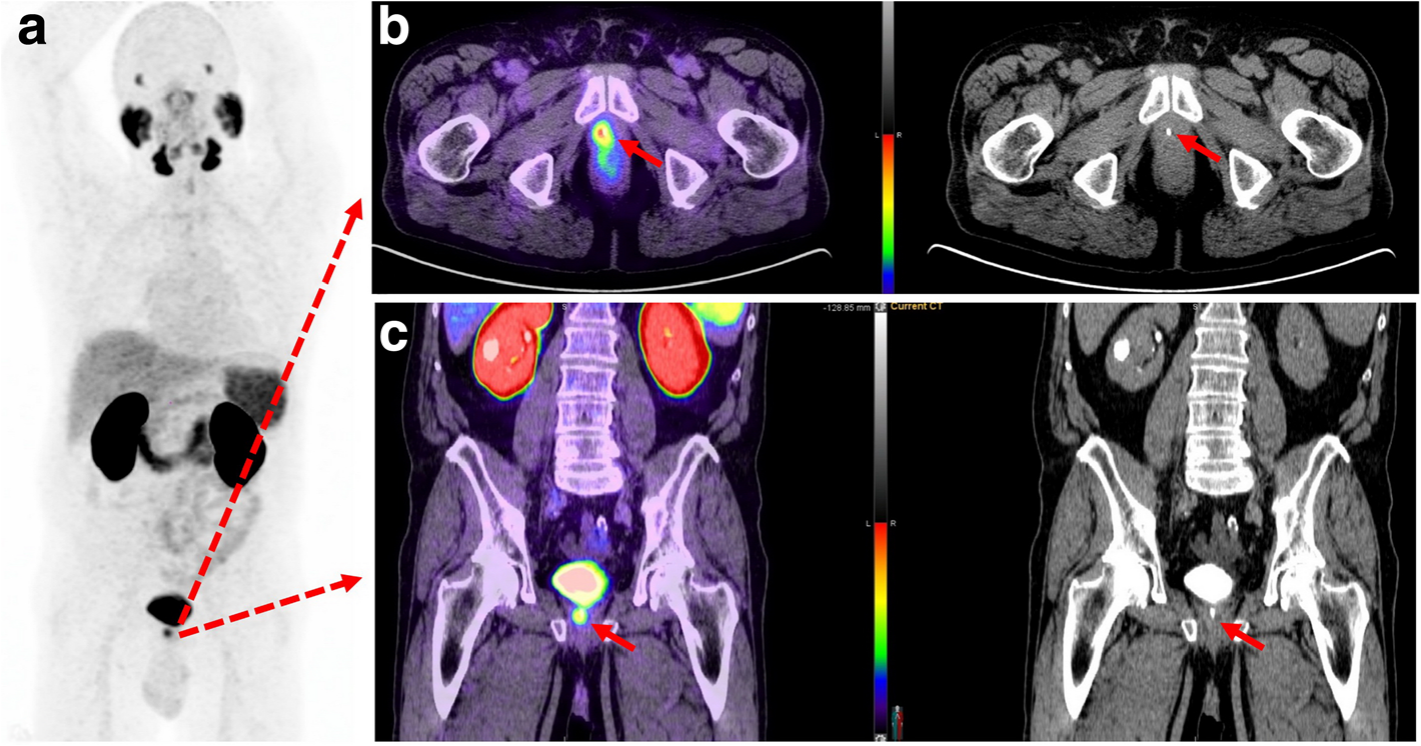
Seventy-six-year-old man with BCR and PSA 5.7 ng/L following prostatectomy. Panel a. PSMA PET MIP image shows focal activity in the prostatectomy fossa. Panel b, c. PET/CT-U and CT-U axial (top row) and coronal (bottom row) show focal activity corresponding to contrast material in the urethra-vesical junction. No PSMA-avid site of recurrence was detected in this patient
